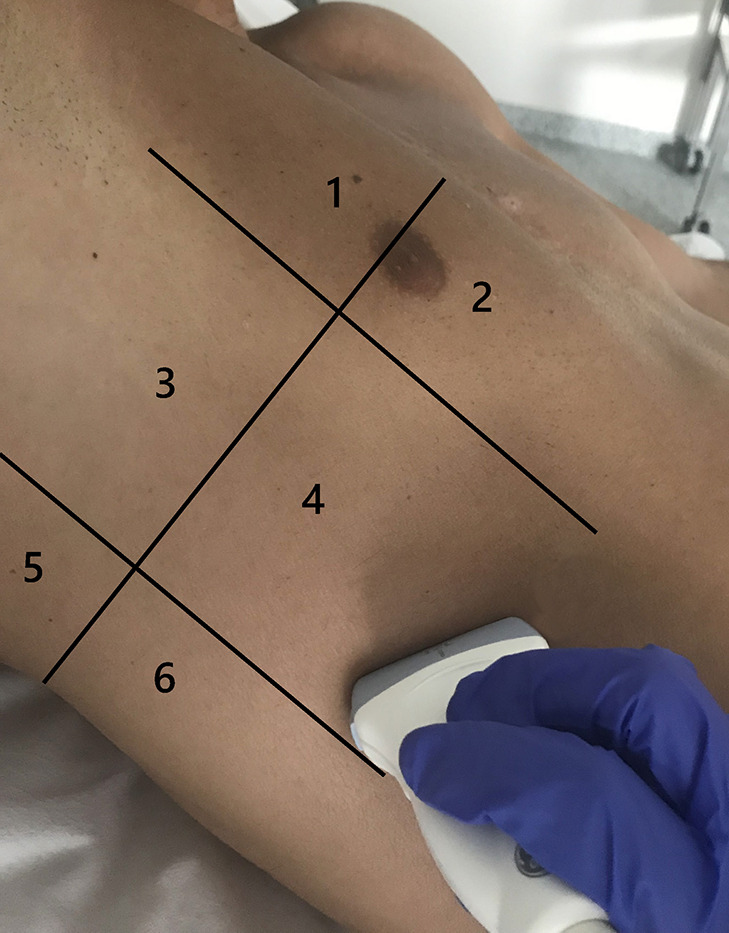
Lung regions scanned in a 12-region LUS exam shown for one hemithorax. LUS images were acquired using a linear transducer and a transversal approach. Zones 1 and 2 are anterior LUS regions, zones 3 and 4 are lateral LUS regions, and zones 5 and 6 are posterior LUS regions. LUS, lung ultrasound.
